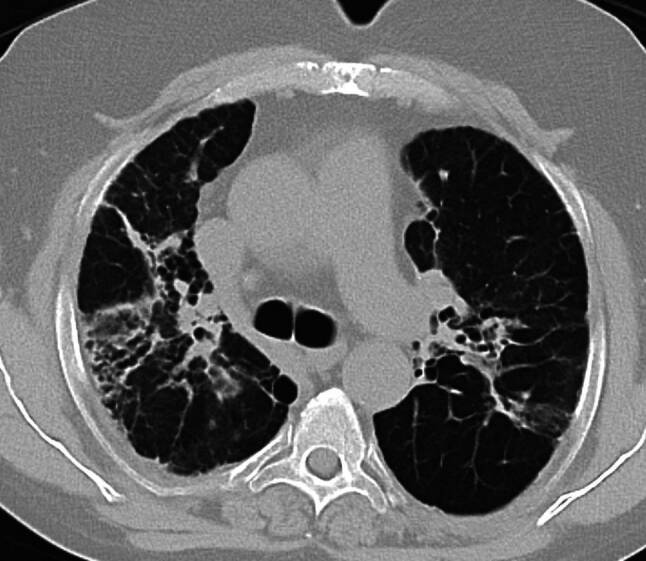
CT-morphologisches Bild einer SAPF mit bronchozentrischer Betonung, Bildung von Traktionsbronchiektasen und Volumsreduktion. (Quelle: KUK Linz, Universitätsklinik für Innere Medizin 4 – Pneumologie)
